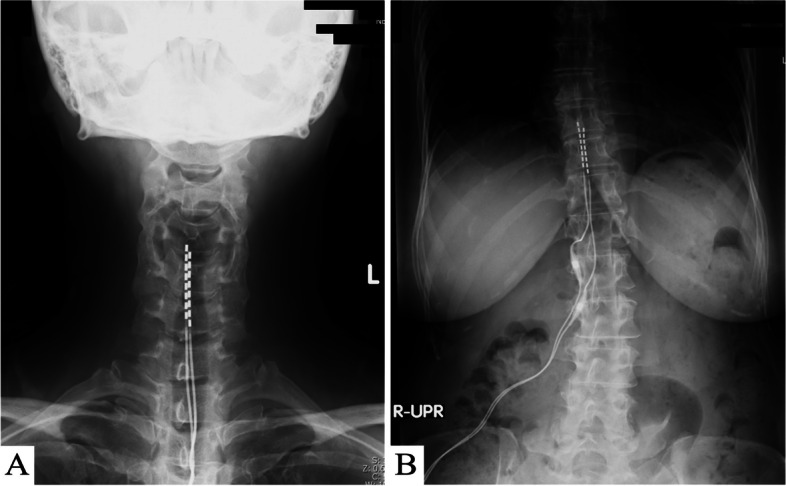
Radiograph of case 2 after SCS placement. A Cervical radiograph after SCS implantation. B Thoracic radiograph after SCS implantation
